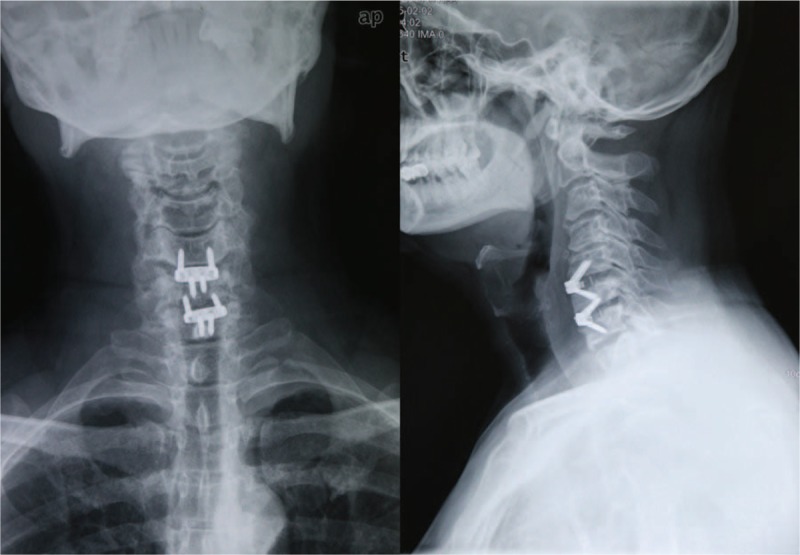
Three months postoperative x-rays showed a good position of the implant.
